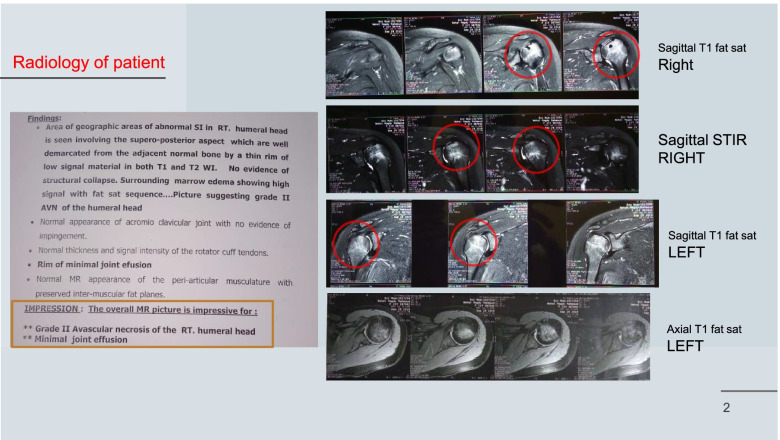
MRI of both shoulders revealed abnormal signals in the right humeral head involving the superior-posterior aspect which are well demarcated from the adjacent normal bony by a thin rim of low signal material in both T1 and T2 WI. No evidence of structural collapse with marrow edema showing high signal with fat sat sequence. *Conclusion:* grade II AVN of the right humeral head and grade I AVN of the left humeral head with minimal joint effusion
